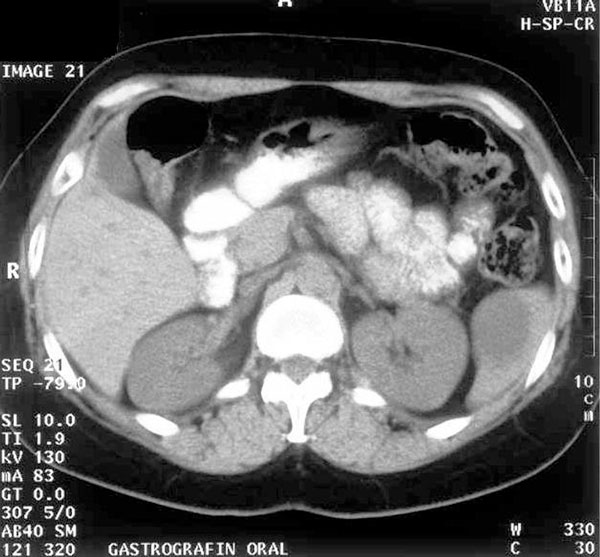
CT scan (1997): A 6 cm splenic cyst is evident at the lower pole of the spleen.
